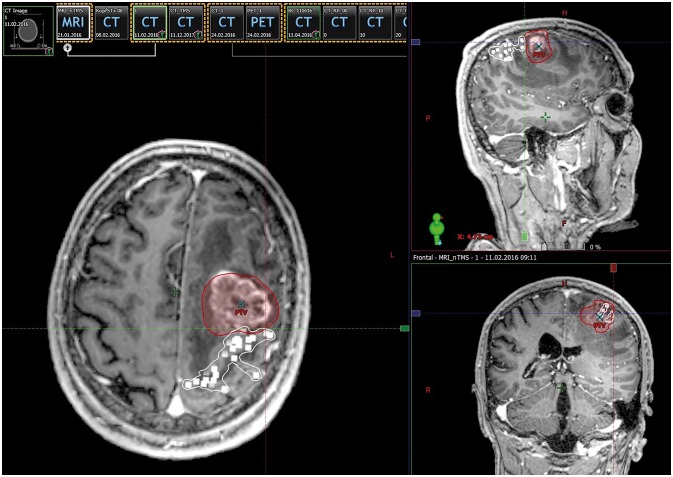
Integration of motor maps in target volume delineation. This figure shows contrast-enhanced, T1-weighted magnetic resonance imaging (MRI) fused with navigated transcranial magnetic stimulation (nTMS) motor-positive points (white squares) in an exemplary patient case. For radiotherapy (RT) planning, nTMS motor maps were contoured as coherent organs at risk (OARs) in terms of target volume delineation. The planning target volume (PTV) is depicted as a red area.
